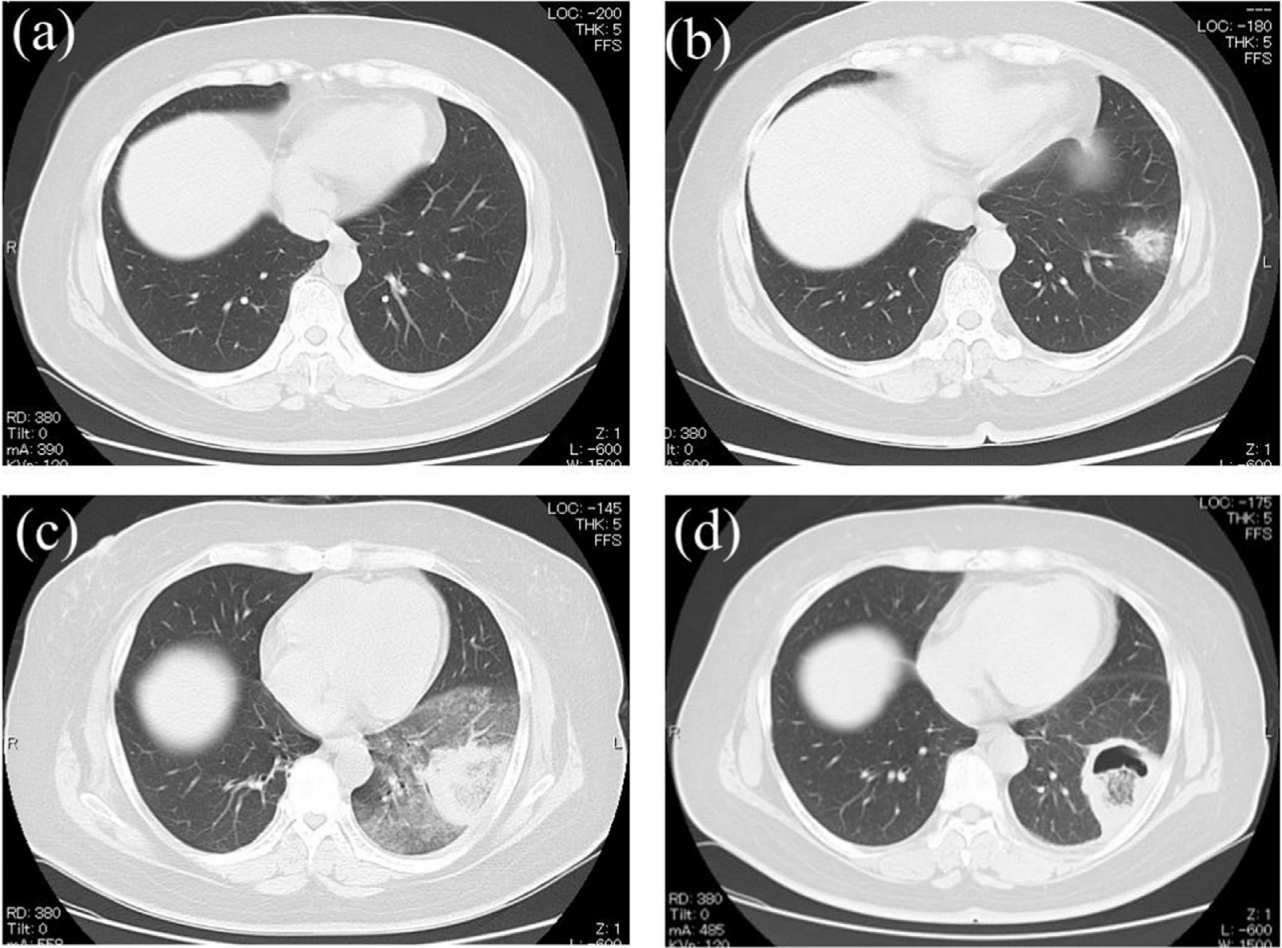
CT findings. (a) No abnormal shadow was observed at day + 47. (b) Halo sign observed at day + 54. (c) Reversed halo sign observed at day + 61. (d) Mass shadow with cavity observed at day + 89.
